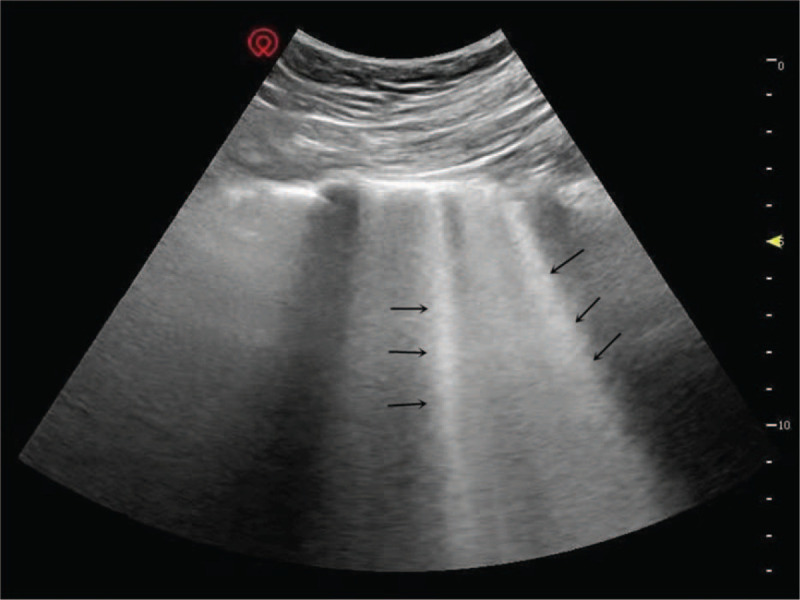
B lines were detected by ultrasound (black arrows).
